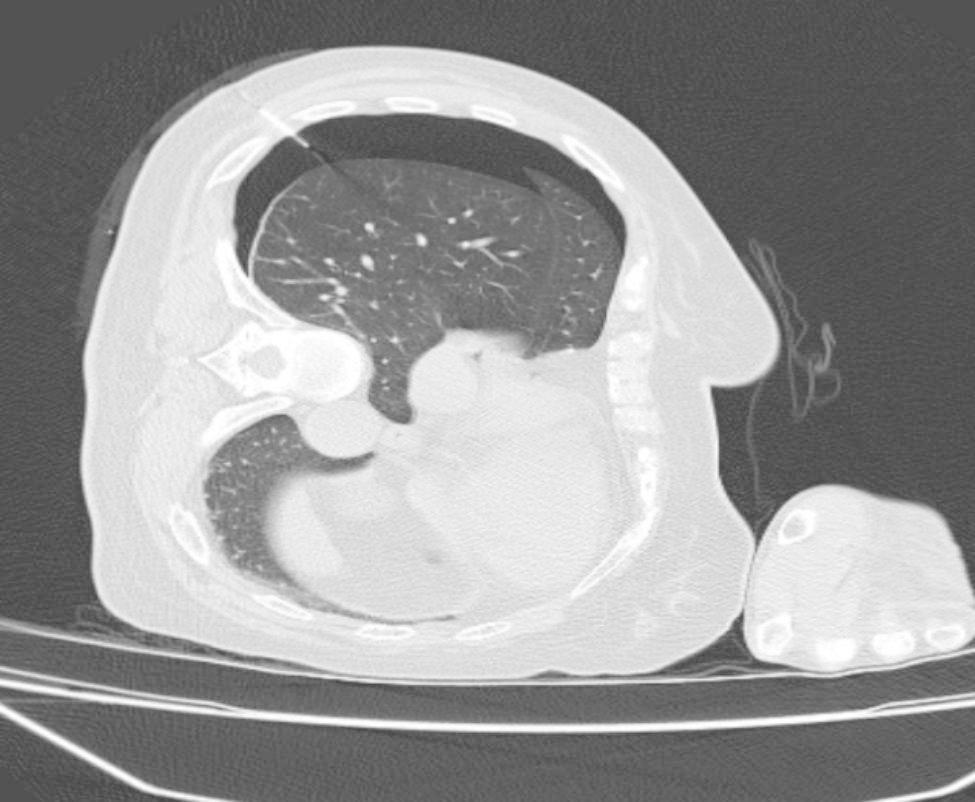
Images of lung CT scan for the development of pneumothorax. A mild-sized right pneumothorax, characterised by a banded area of very low density without lung texture, compression of the lung parenchyma and deviation of the mediastinum toward the contralateral hemithorax
